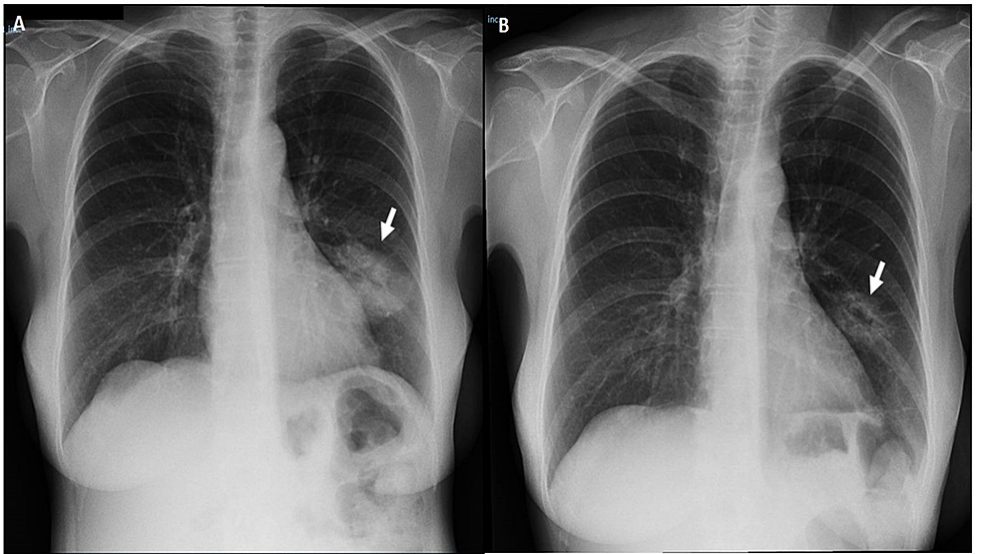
X-ray showing typical plain radiographic findings of aspergilloma A: The first X-ray assumed as a bacterial pneumonia showing a intrapulmonary soft tissue-like mass B: X-ray after 23 days showing a crescentic lucency surrounding the peripheral aspect of the mass
